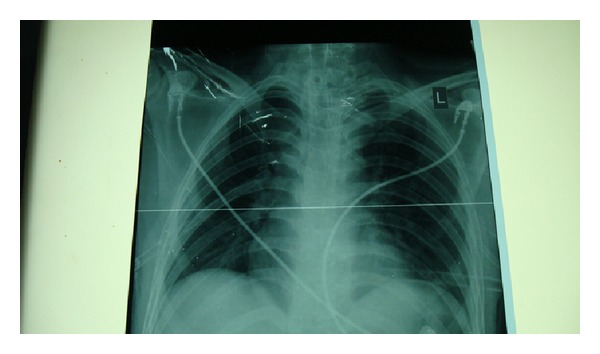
X-ray chest showing tracheostoma in the stent with bilateral chest drains *in situ*.
